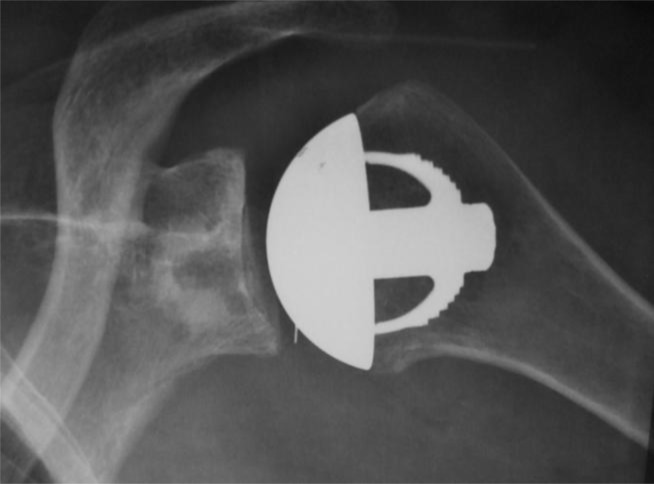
A–F: Postoperative X-ray: cemented stemmed humeral prostheses (A), TSA with uncemented humeral component and cemented all-polyethylene glenoid component (B), TSA with TMT glenoid component (C), TSA with metal-backed glenoid component (D), humeral resurfacing (E), uncemented stemless shoulder prostheses with cemented polyethylene glenoid component (F).
